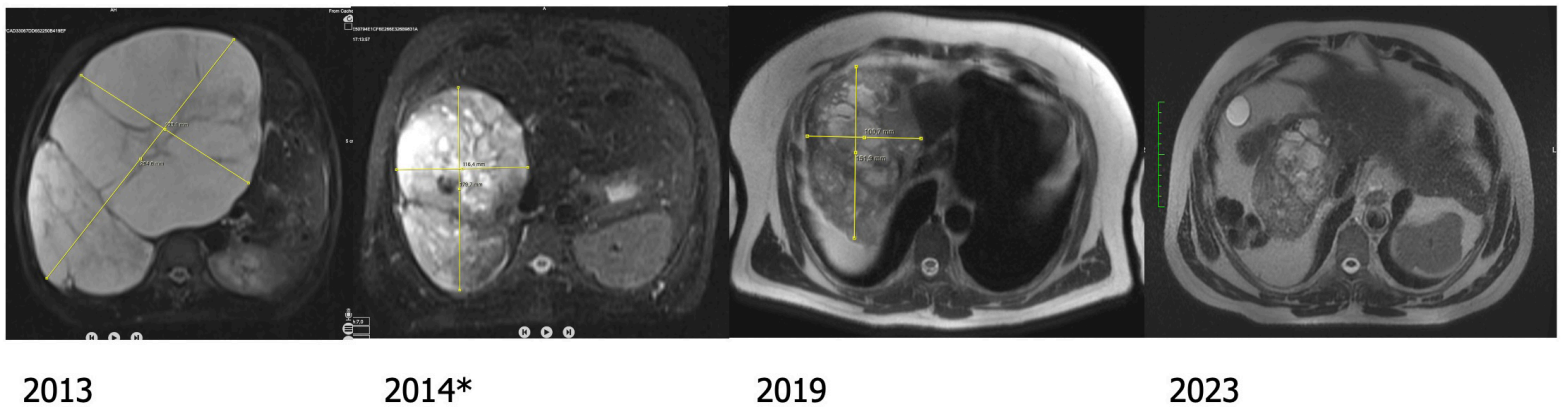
The patient underwent two planned TACE sessions. The MRI taken 6 months after the second session* shows significant volume regression which progressed even after 5 years.
